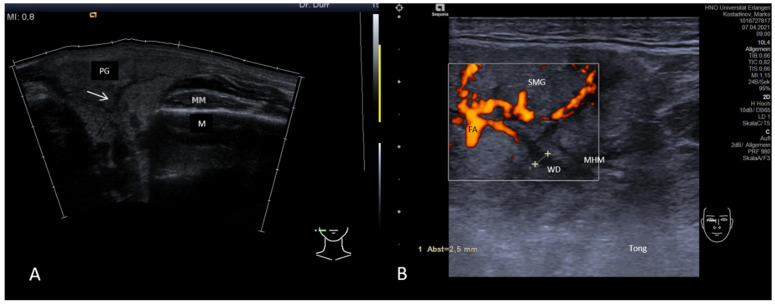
Acute viral sialadenitis in the PG (A) and in the SMG (B). The volume of the gland shows a tendency towards enlargement. The parenchyma is hypoechoic mainly by a spongy aspect (white arrow), which is caused by duct dilation and increased perfusion, which cannot be distinguished by plain US. Doppler-coded sonography was used to differentiate between duct dilation (Wharton’s duct dilation measuring 2.5 mm, dotted line) and vascular structures (low-flow perfusion indicated by Power Doppler, B). Abbreviations: PG, parotid gland; SMG, submandibular gland; M, mandible; MM, masseter muscle; MHM, mylohyoid muscle; Tong, tongue; WD, Wharton’s duct; FA, facial artery.
